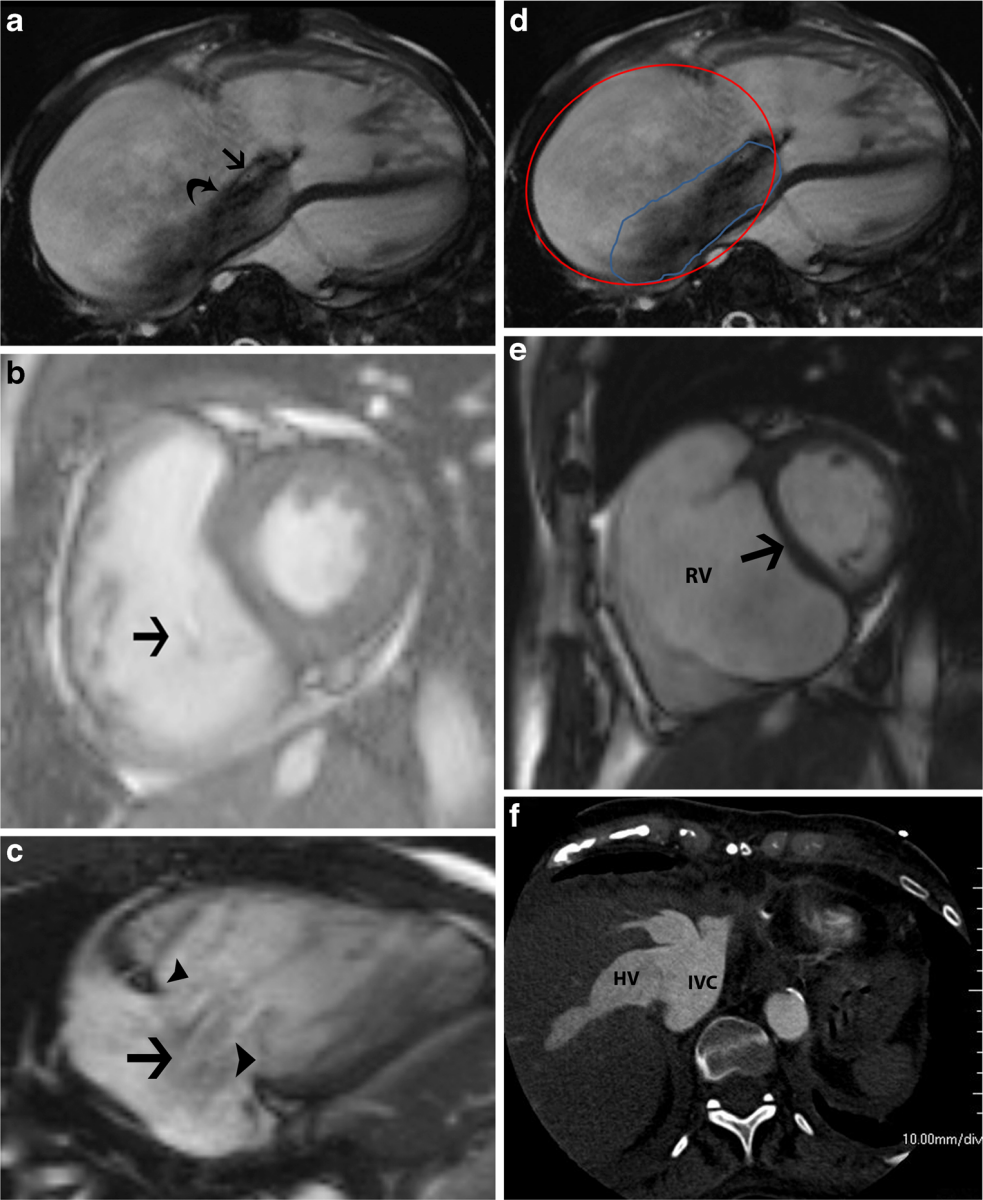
Quantification of tricuspid regurgitation (TR). (a) Vena contracta is the diameter of the smallest regurgitant flow (*straight arrow*) before expansion of the jet (*curved arrow*) in a four-chamber view. (b) Effective regurgitant orifice is measured from a short-axis cine steady-state free precession (SSFP) image through the tricuspid valve in systole. (c) Failure of coaptation of leaflets in four-chamber cine image (*arrowheads*) in a patient with significant TR (*arrow*). (d) Jet area (*blue*) and jet area/left atrial area are measured in a four-chamber SSFP or phase-contrast image. TR grade: mild > 5 cm^2^, moderate 6–10 cm^2^, severe >10 cm^2^. Ratio of regurgitant area to RA area: mild > 20 %, moderate 20–34 %, severe > 35 %. (e) Short-axis steady-state free precession (SSFP) image through the ventricles shows a flat ventricular septum (*arrow*). (f) CT image through the liver shows reflux of contrast through the hepatic veins and IVC
